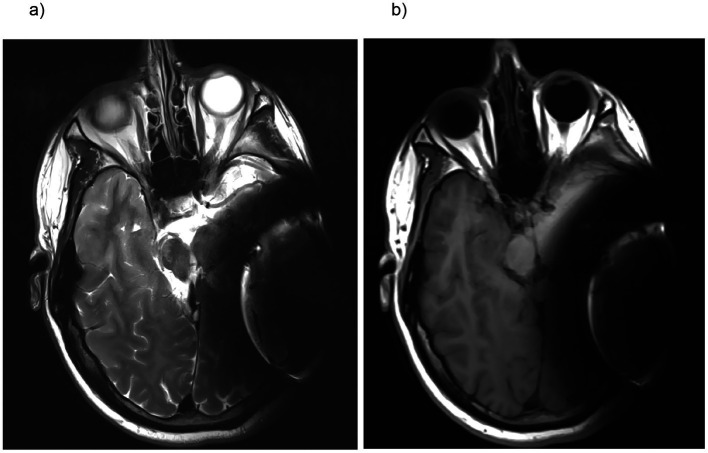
MRI (magnetic resonance imaging) in T2-weighted sequence (a) and T1-weighted turbo inversion recovery magnitude (TIRM) images (b) in a unilateral CI with a magnet positioned at a 120° angle. As shown, both the contralateral and ipsilateral orbits are fully visible and assessable.
